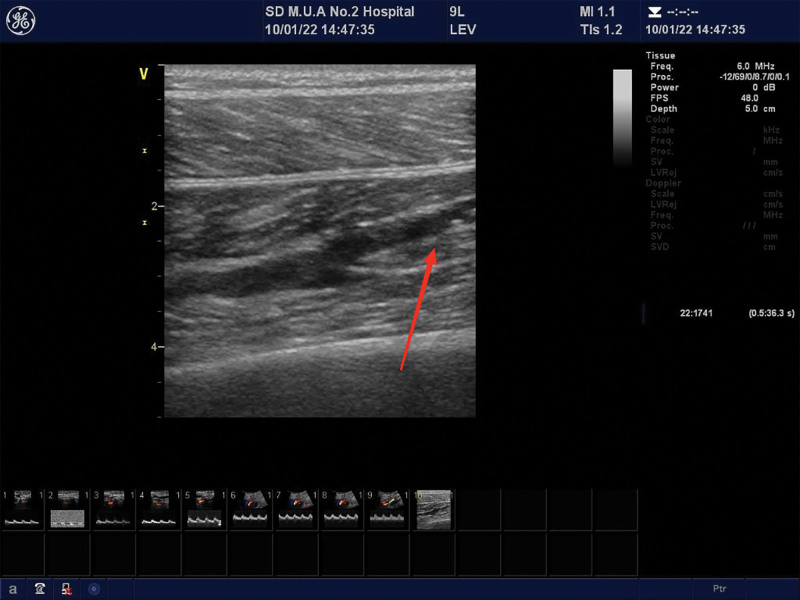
Visualized representation of MCVT in 2 patients were listed in Figure 2. The Red Arrows indicate MCVT which was diagnosed by vascular ultrasonography. MCVT = muscular call vein thrombosis.
